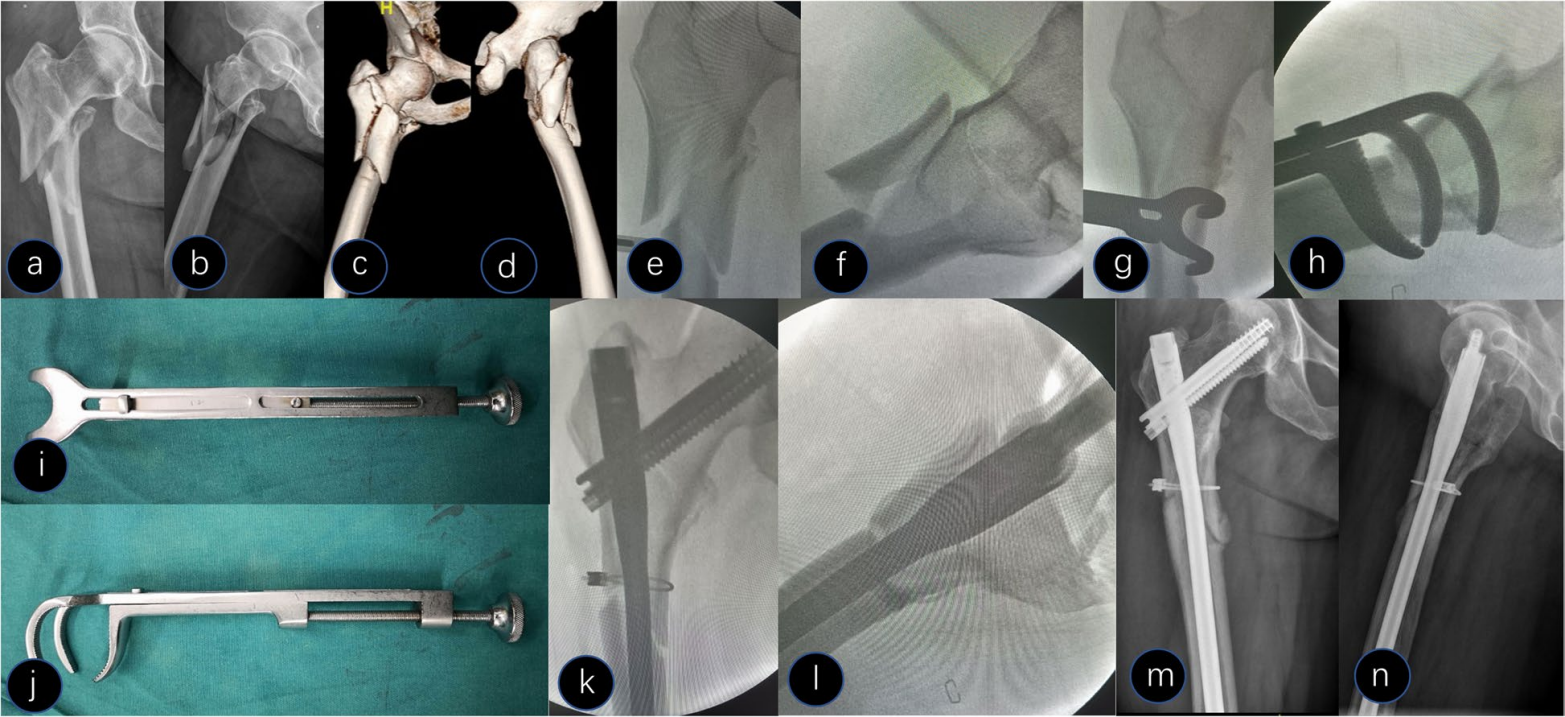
A 54-year-old female presented with a Type V fracture. a-d Preoperative X-ray and computed tomography 3D reconstruction showed medial-lateral displacement of the subtrochanteric region. e and f Closed reduction with traction under fluoroscopy. g and h A Lowman clamp was used to reduce the subtrochanteric fracture during the operation. i and j Pictures of the Lowman clamp. k and l Intraoperative X-ray after reduction and fixation (a cerclage cable was used to fix a large butterfly-shaped fragment). m and n Postoperative X-ray at 6 months
